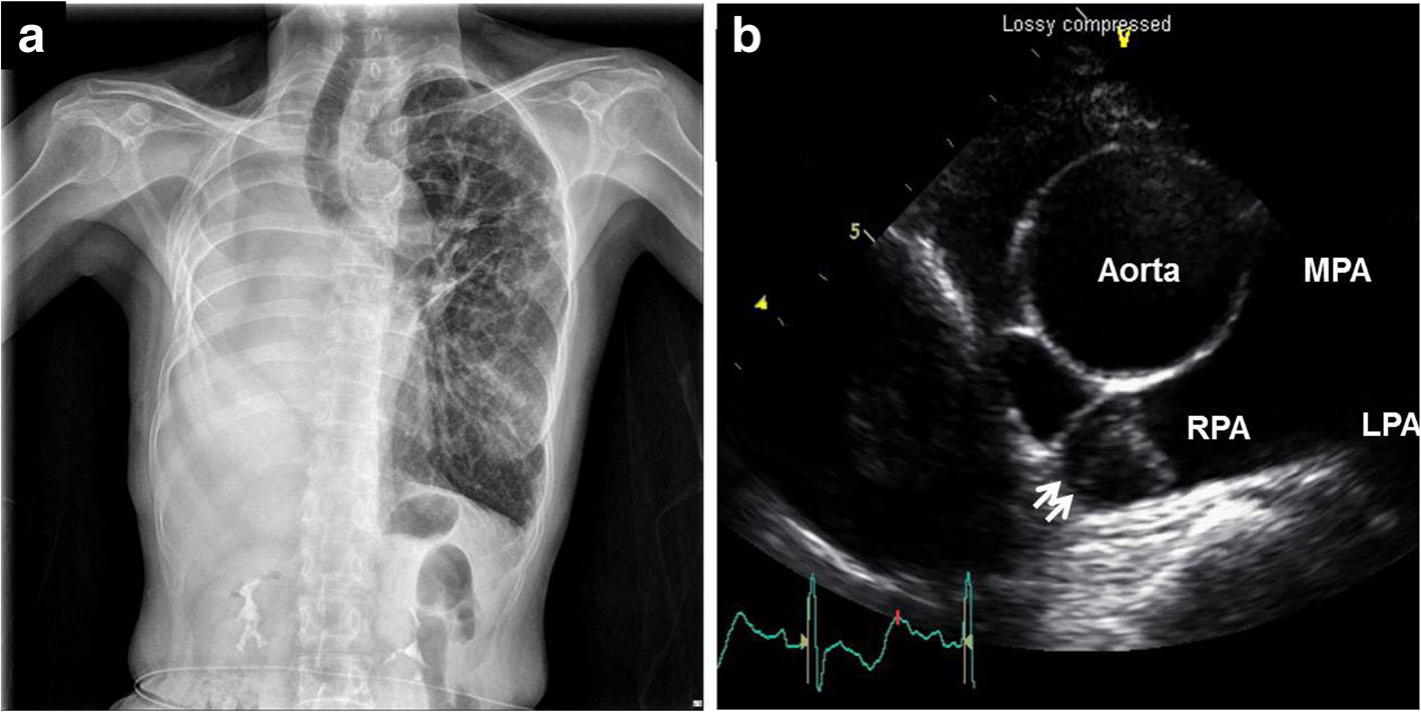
Chest X-ray showing total hazziness on right lung field due to previous pneumonectomy (a). Echocardiography demonstrating about 1.7 × 1.5 cm sized round echogenic mass within the dilated stump of the right pulmonary artery (RPA) (b). MPA: main pulmonary artery. LPA: left pulmonary aretry
